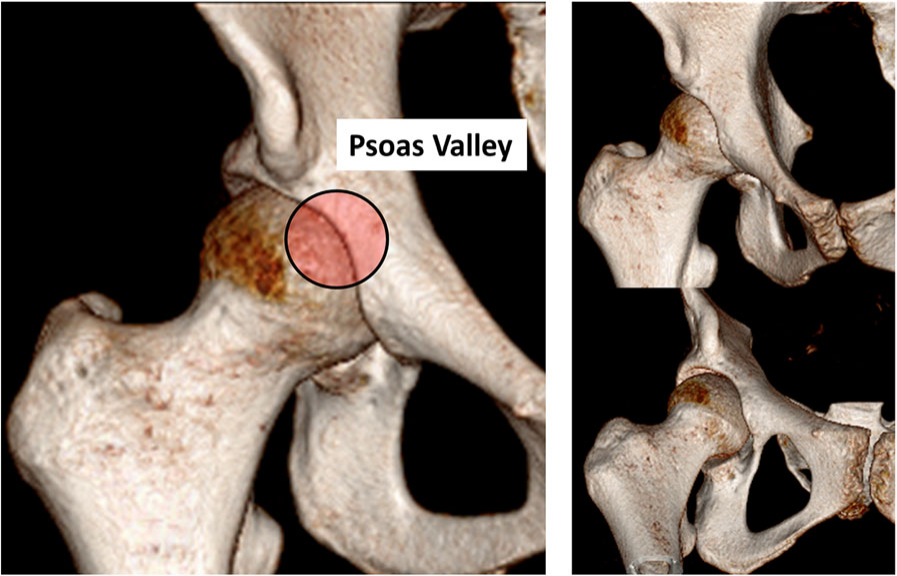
Psoas valley in 3D-CT scan
